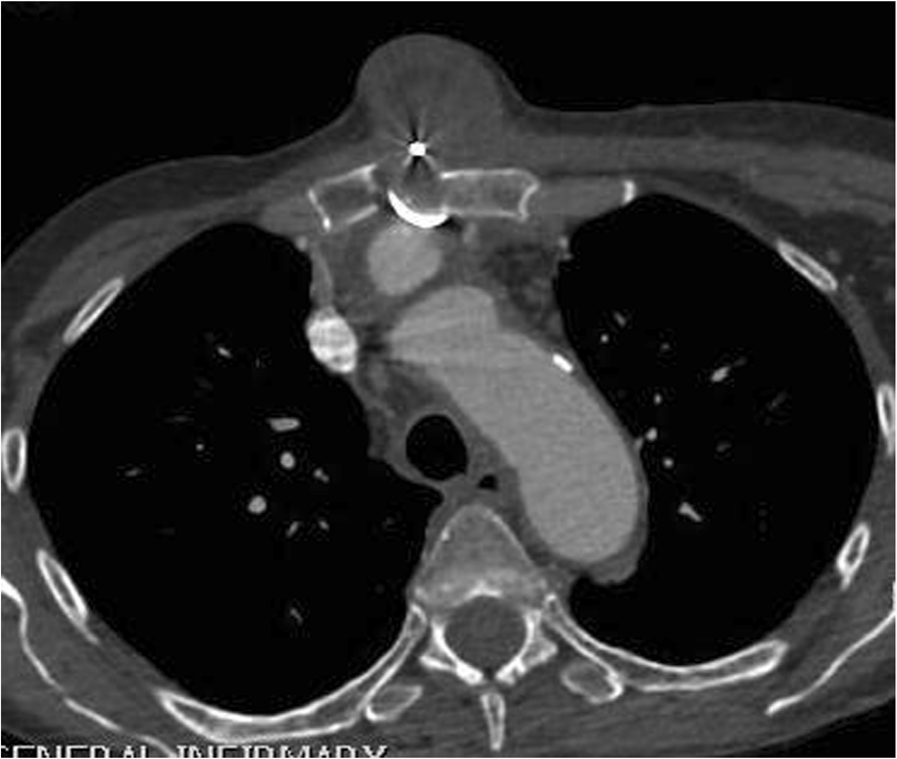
CT scan confirms subcutaneous collection overlying manubrium, manubrial disruption, posterior dislocation of the sternal wire which is seen to lie close to the innominate graft.
